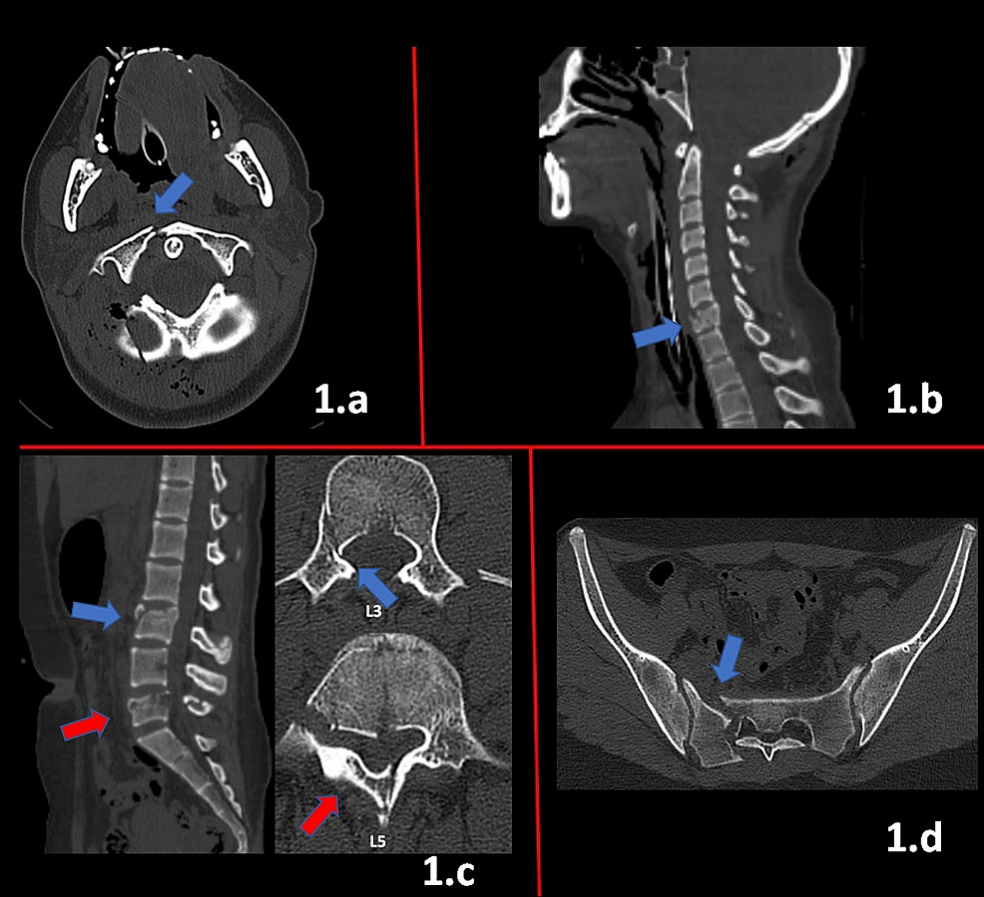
a) cervical CT axial view: right C1 anterior arch fracture (blue arrow), b) cervical CT sagittal view: C7 burst fracture (blue arrow), c) lumbar CT sagittal and axial views of L3 (blue arrow) and L5 burst fractures (red arrow), d) sacrum CT axial view: Denis zone 2 sacral fracture (blue arrow)
